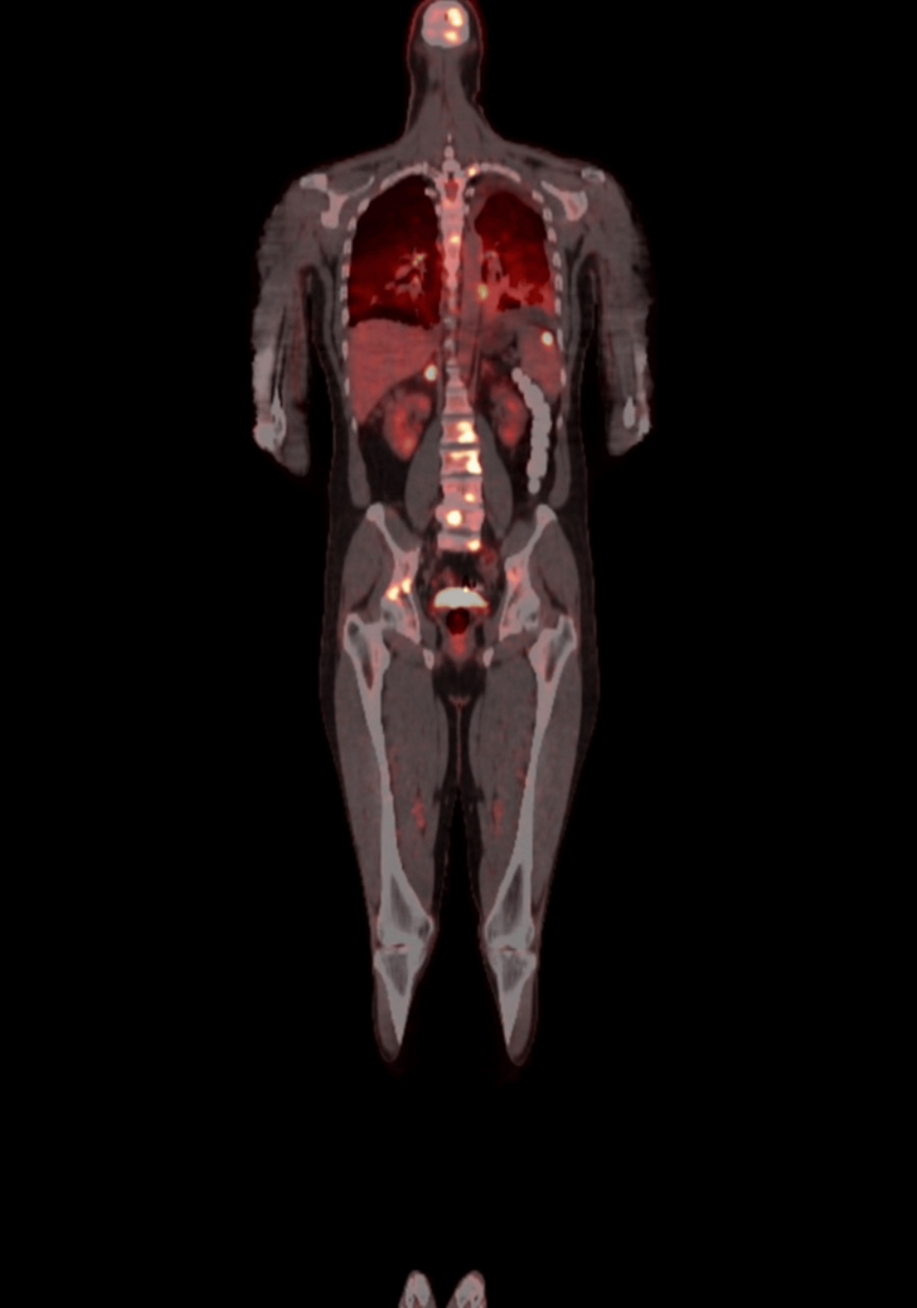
PET scan The scan demonstrates FDG-avid lesions involving the brain, mediastinal and hilar lymph nodes, lungs, spleen, right adrenal gland, liver, and multiple bones, consistent with widespread metastatic disease. PET: positron emission tomography; FDG: fluorodeoxyglucose
